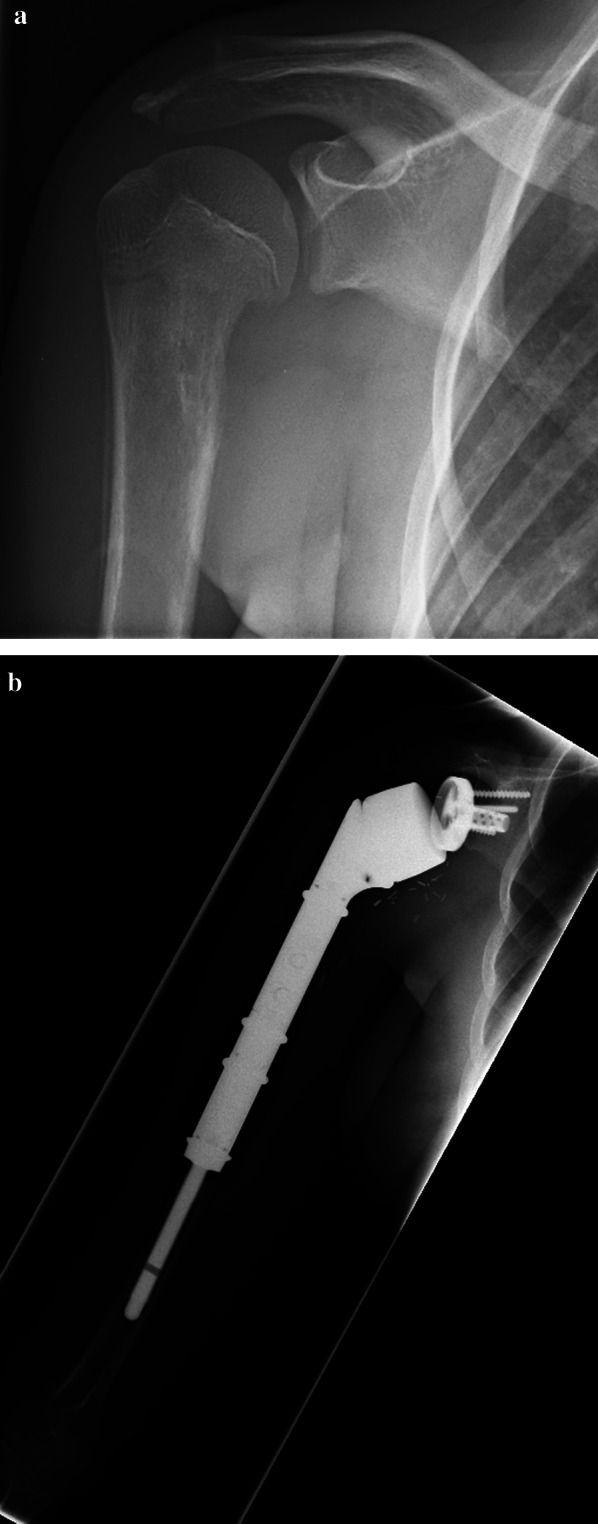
a Osteosarcoma of the right proximal humerus in an 18-year-old female patient; b radiographic imaging 40 months following PHR
